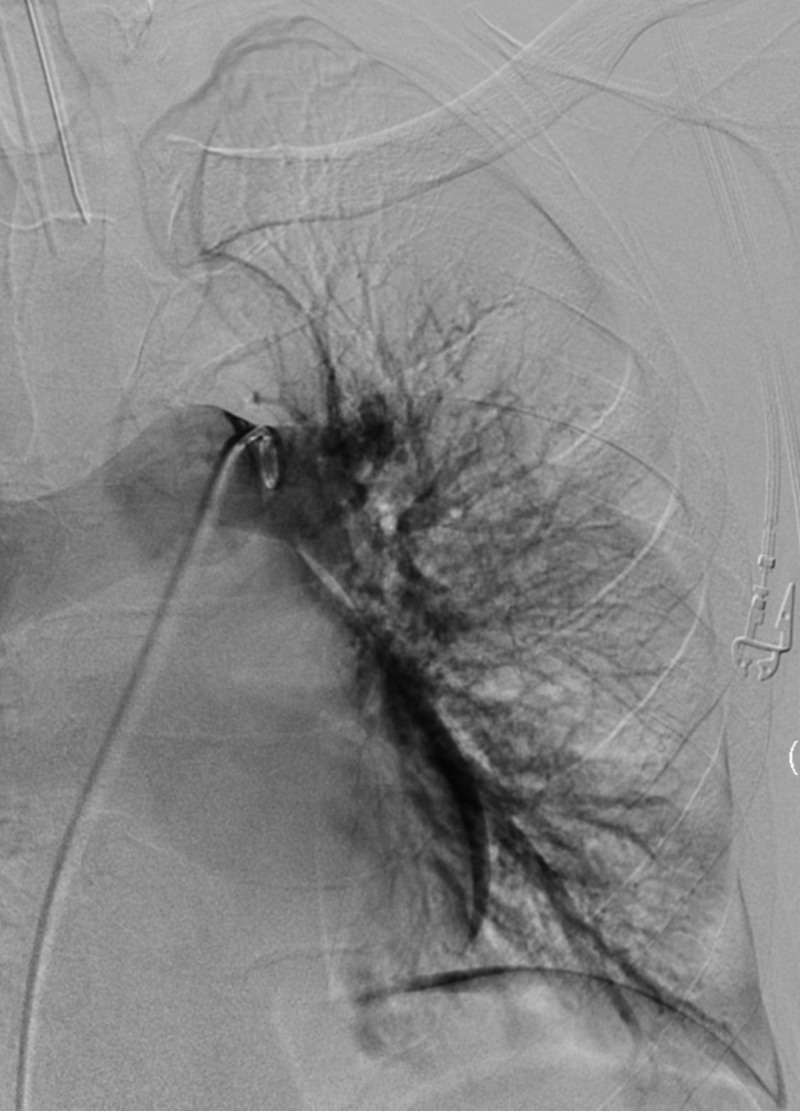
Left Lung Post-Mechanical Embolectomy CT Angiography Showing Improved Perfusion to the Upper and Lower Lobes
